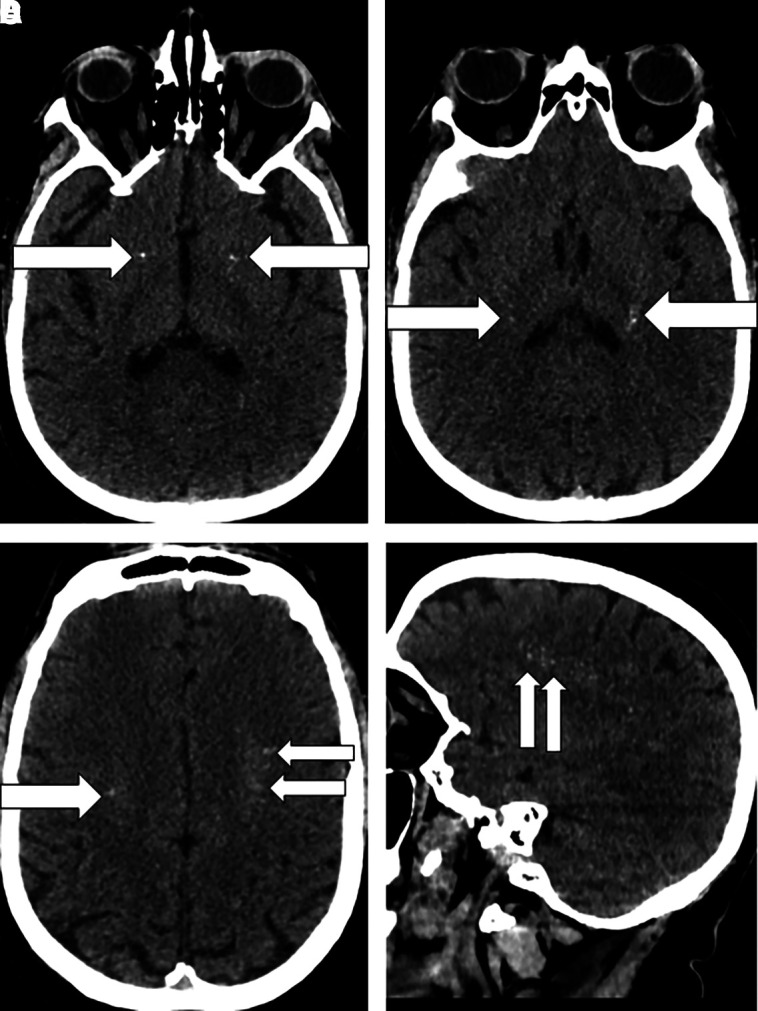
Brain CT scan showed bilateral asymmetrical punctate calcifications in the basal ganglia. Panel A, axial image with putamen calcifications (arrows). Panel B, axial image with calcifications in the posterior limb of the internal capsule (arrows). Panel C, axial image with bilateral and asymmetrical punctate and linear calcifications in the bilateral corona radiata (arrows). Panel D, sagittal image with punctate and linear calcifications in the left corona radiata (arrows).
